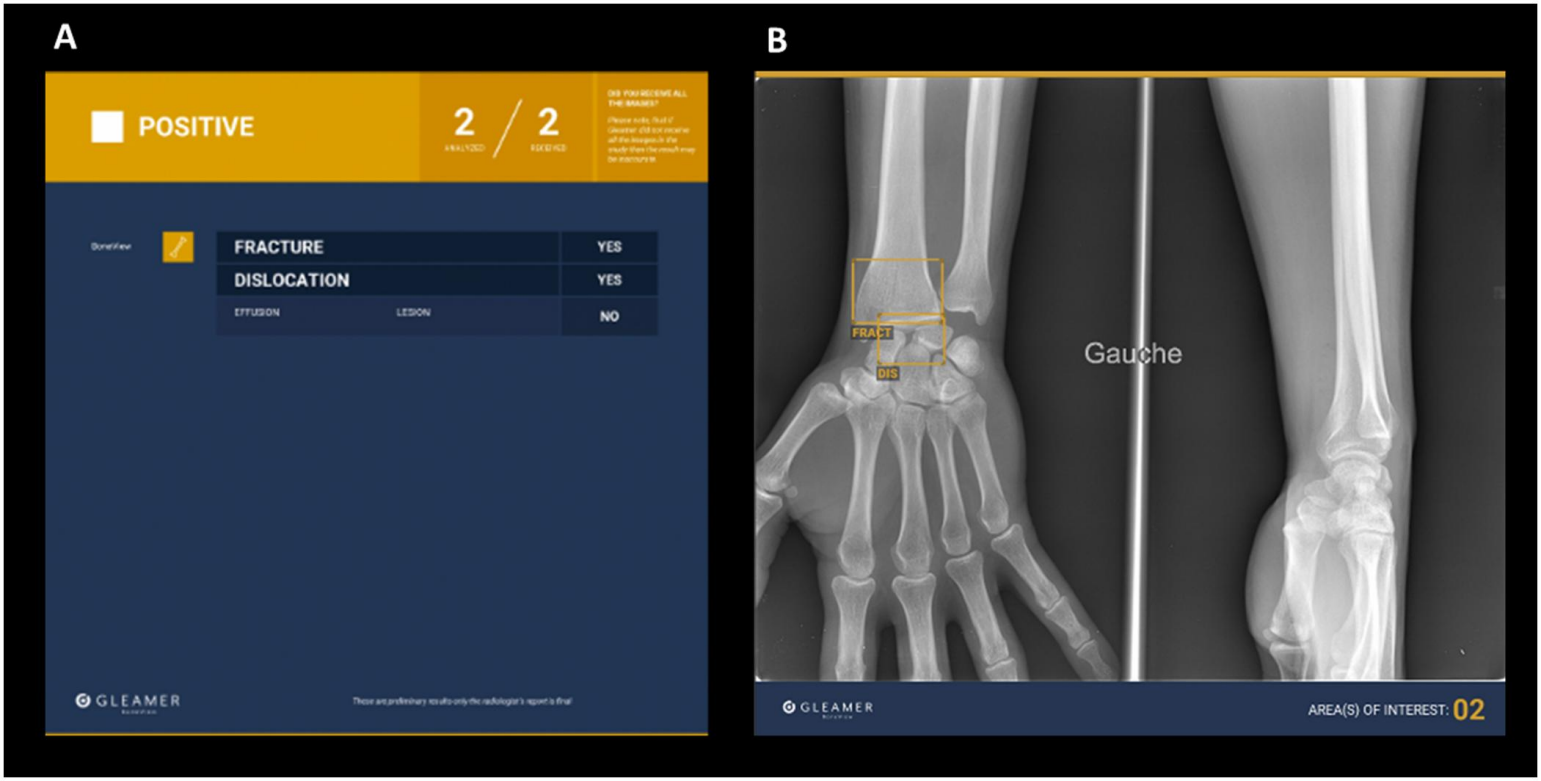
This image demonstrates the results produced when using an artificial intelligence (AI) fracture detection tool by Gleamer, called BoneView. With this product, a summary image is sent to PACS, depicted in figure (A) which shows the number and type of pathologies detected on the radiograph. (B) A second image is also sent to PACS with bounding boxes and their associated labels (FRACT = fracture, DIS = dislocation) displayed as an “overlay” across the original radiographic image in question. In this example, the AI has flagged a fracture of the distal radius, with a scapholunate dislocation in a child. Image provided by Daniel Jones, Gleamer.
